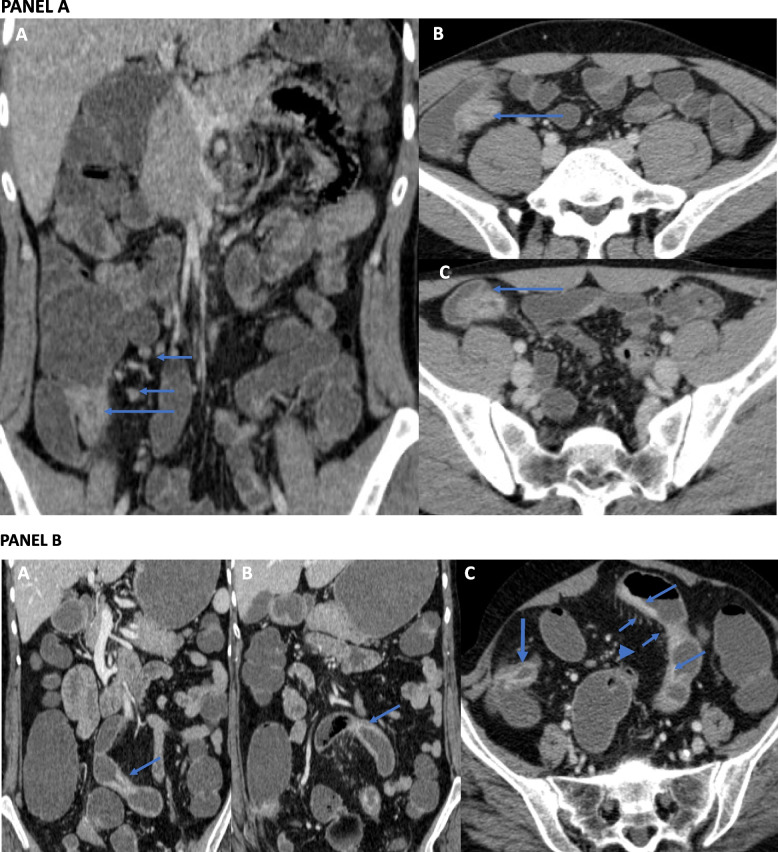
PANEL A: Coronal (A) and Axial (B, C) contrast enhanced CT enterography images show circumferential mural thickening of the terminal ileum and ileocaecal junction (arrows). There are subcentimetric mesenteric lymph nodes (short arrows, A). The patient was diagnosed to have Crohn’s disease. PANEL B: Coronal (A and B) and Axial (C) CT enterography images show multisegmental asymmetric mural thickening of the ileum (arrows). Note the engorged vasa rectae (short arrows, C) and mesenteric fat proliferation (arrow head, C). The terminal ileum is also involved (thick arrow, C) and shows mural stratification. The patient was diagnosed to have Crohn’s disease
